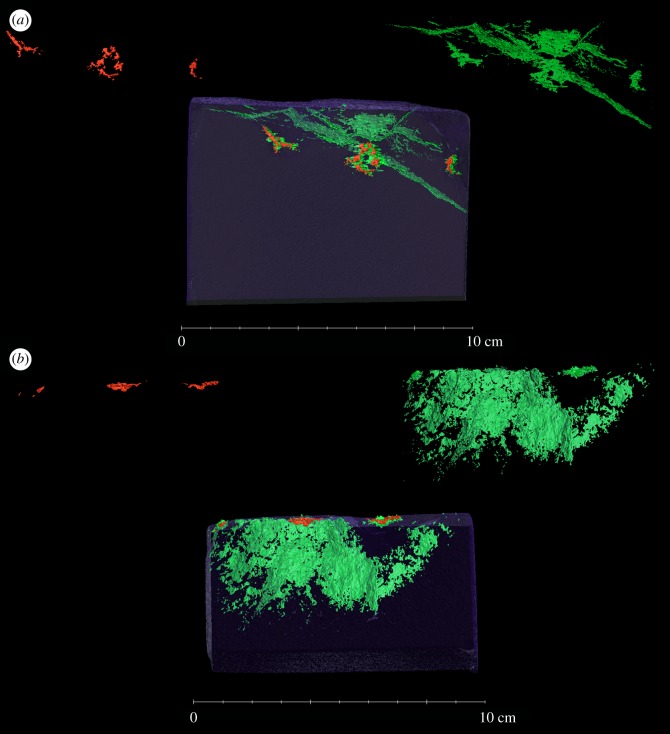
X-ray of impact areas, green demarcates areas of low density (fractured), whereas red indicates areas of higher density, likely to be lead deposits from the bullet encasing. (*a*) Frontal view (line of sight parallel to travel of bullet) and (*b*) top-down view. This sample was not treated with Wacker OH 100.
